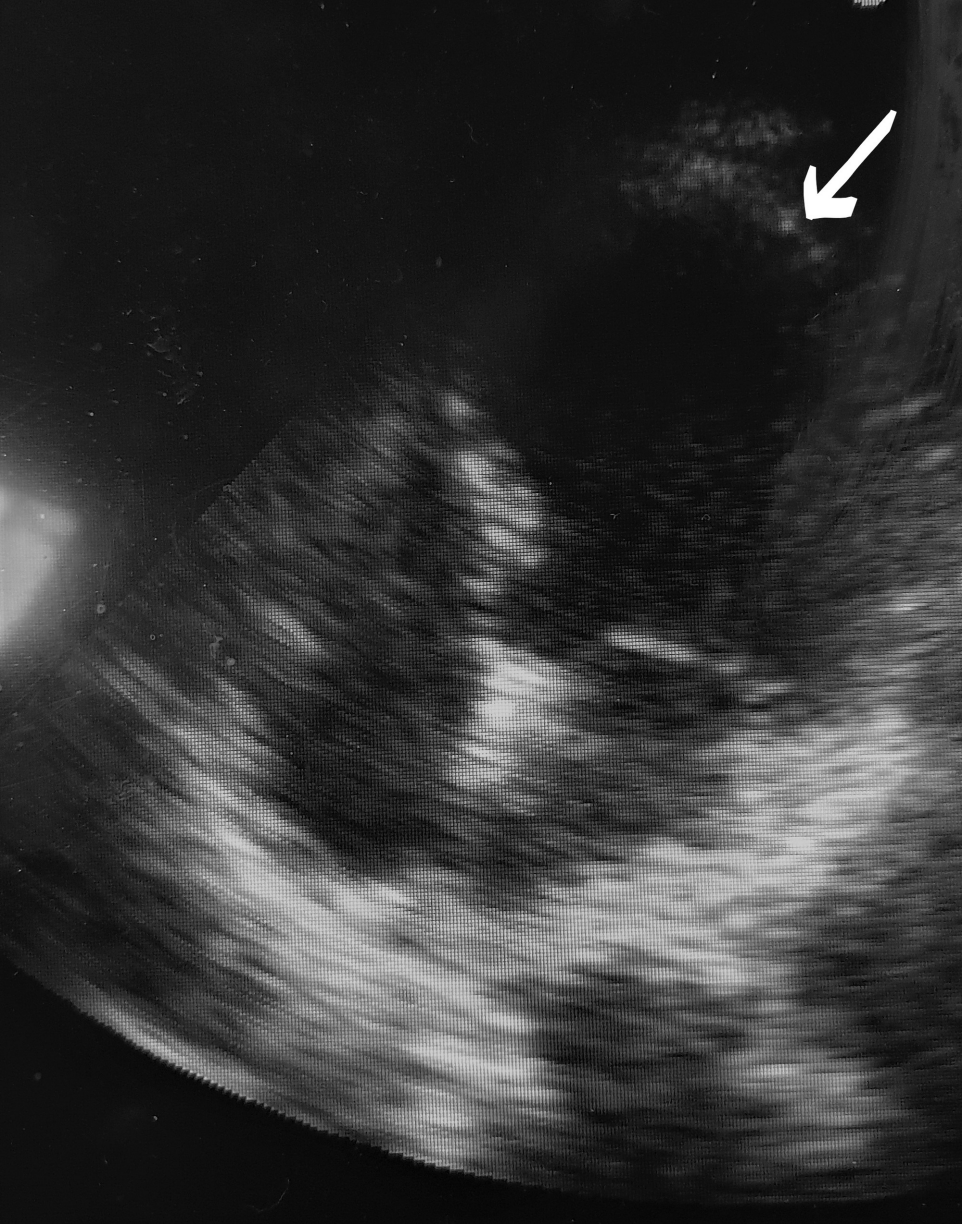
2D Echo showing characteristic left ventricular apical ballooning. 2D Echo, two-dimensional echocardiogram.
